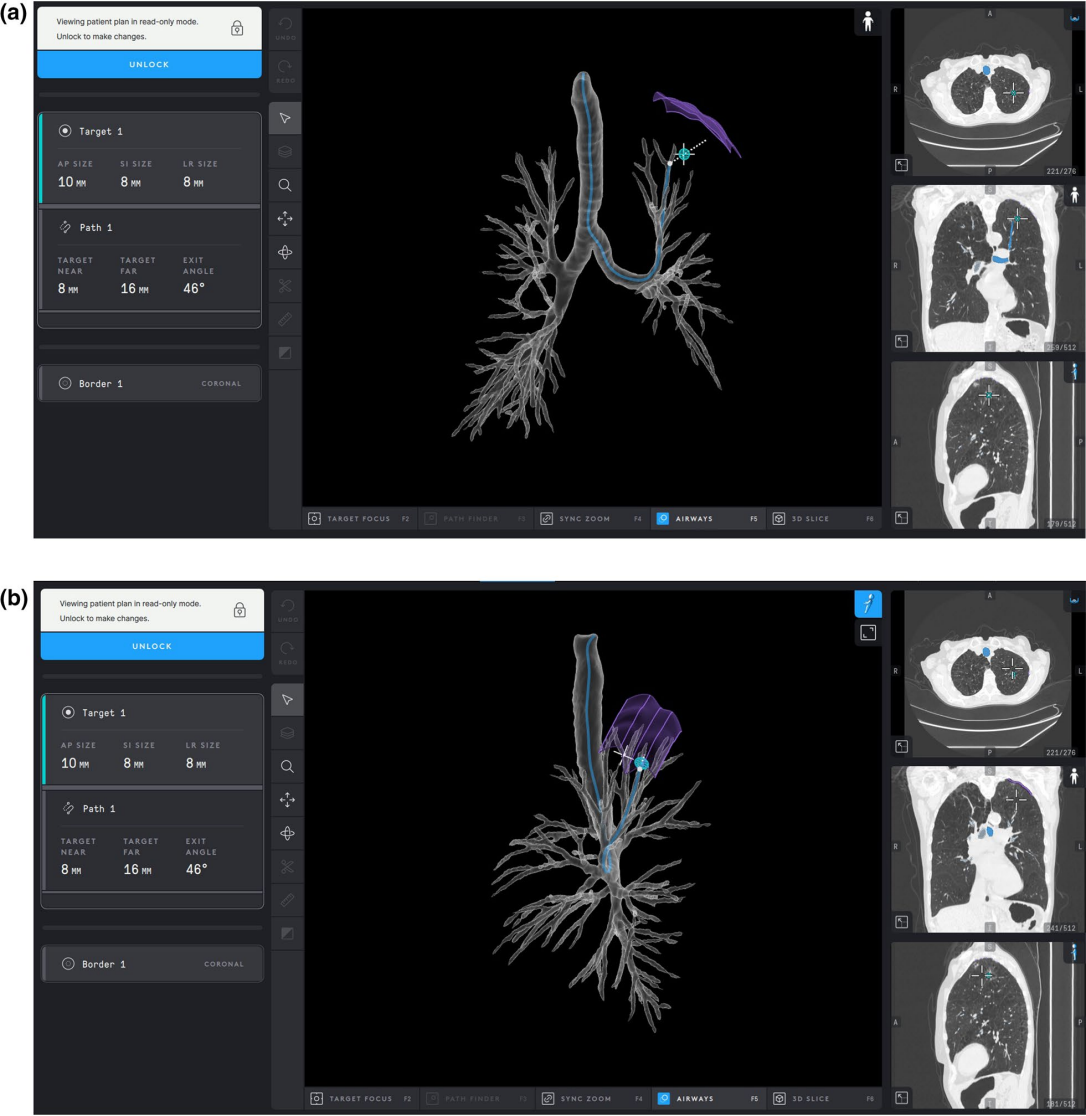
a, b Segmented CT scans of the same subject from PlanPoint planning software from two orientations, both of which show the target nodule, identified pathway, and anatomy border
